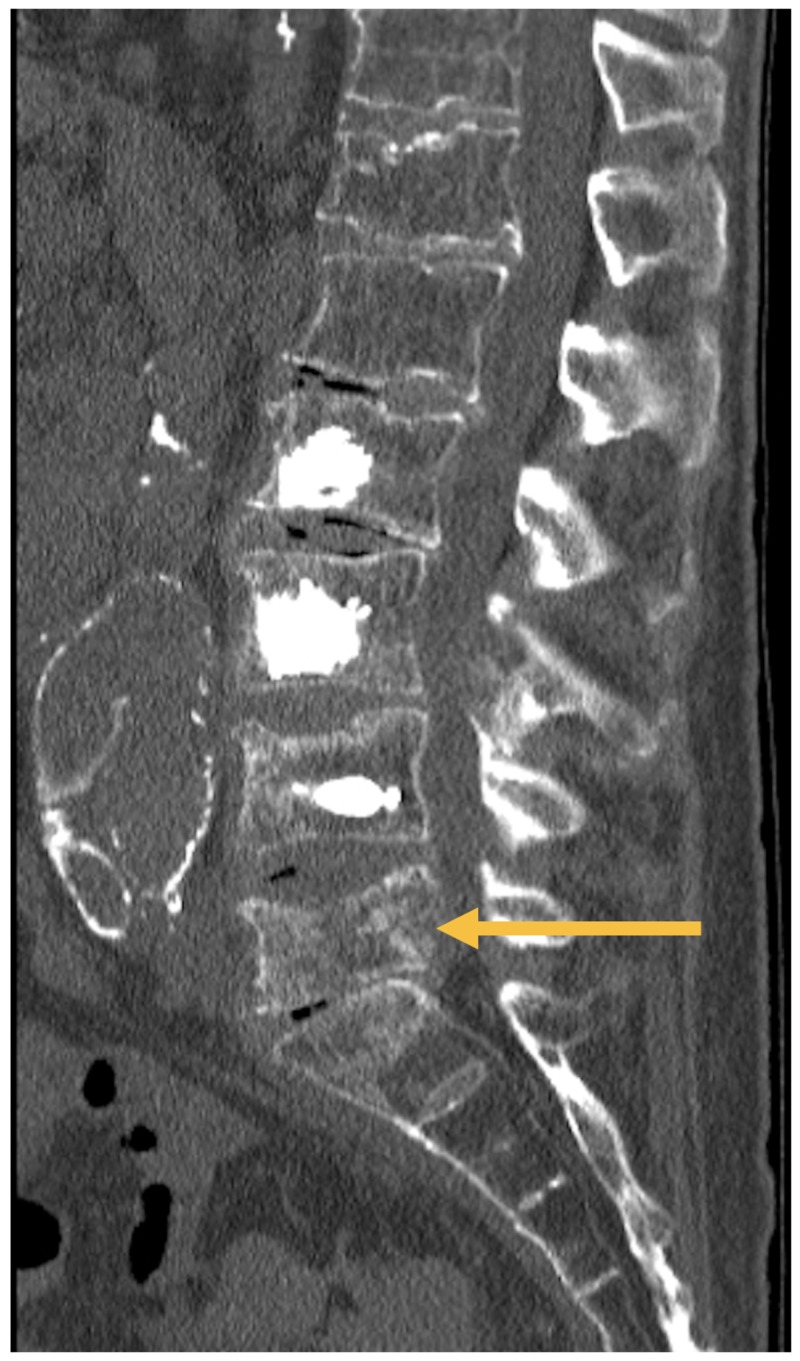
Lateral plain films of the lumbar spine, demonstrating a compression fracture of the L5 vertebral body, with approximately 50% loss of height and mild retropulsion into the spinal canal, and disruption of the posterior endplate. There is also radiographic evidence of prior cement augmentation of the L2, L3, and L4 vertebral bodies.
